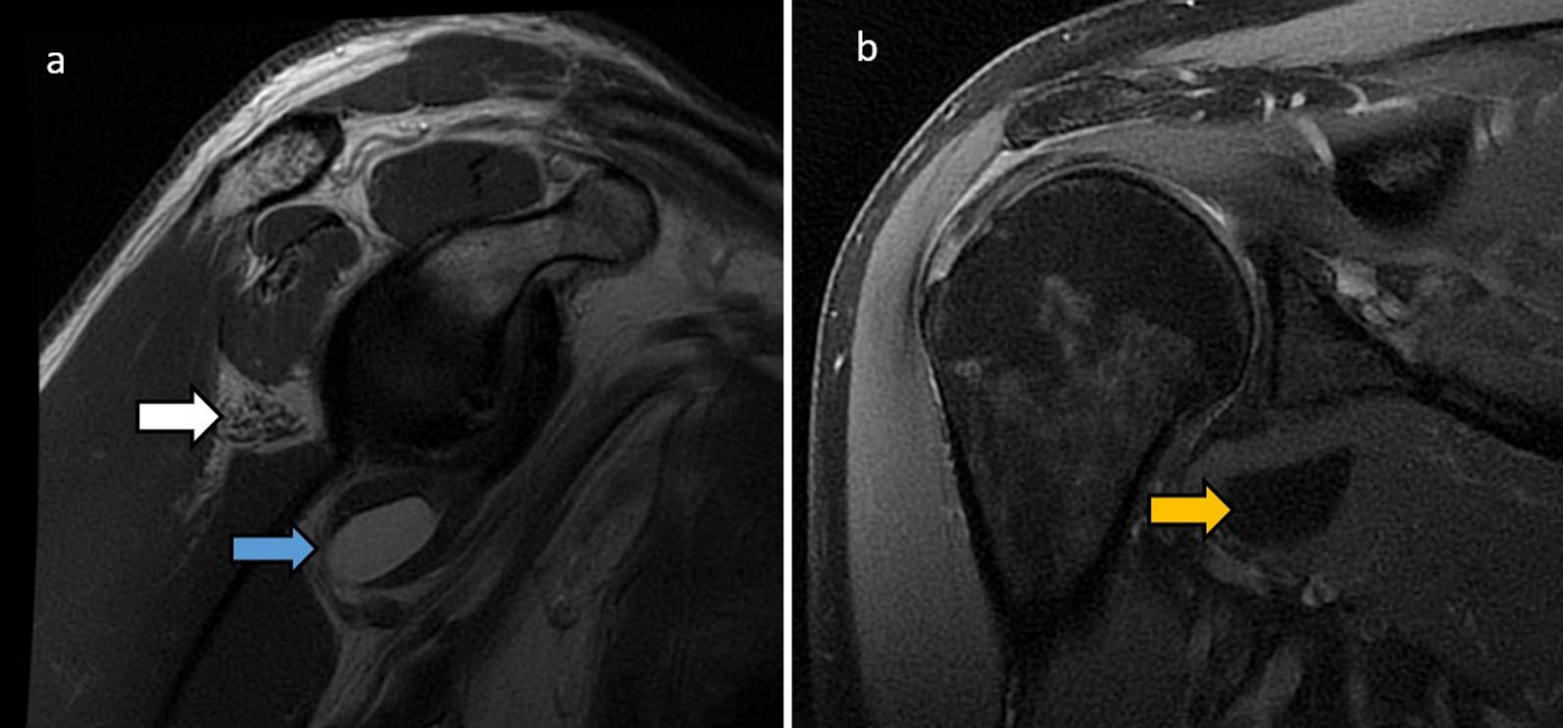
70-year-old with underwent MRI for rotator cuff tendinopathy. Sagittal proton density (PD) shoulder (a) shows severe fatty atrophy of the teres minor (white arrow). There is a lipomatous tumour with complete fat-suppression on the coronal PDFS sequence (b, yellow arrow), which is within the subscapularis muscle belly inferiorly and is impinging on the quadrilateral space and the traversing axillary nerve (a, blue arrow). This is consistent with chronic quadrilateral space syndrome
